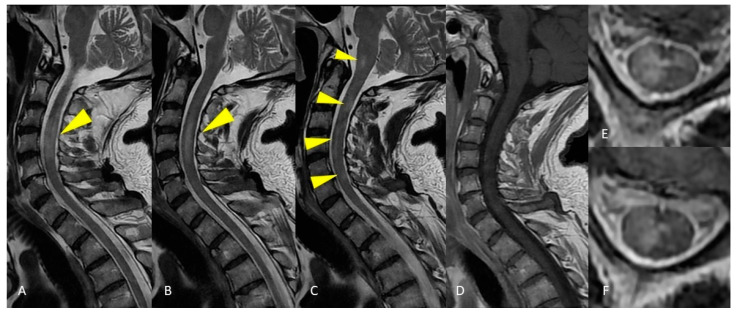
MRI of the cervical spinal cord. Sagittal T2-weighted images of the cervical spine show areas of patchy and long-segment (>1.5 vertebral body length) hyperintensity (A–C) without enhancement on the T1-weighted post-gadolinium acquisition (D). Axial T2-weighted image shows large hyperintensity involving half or more than half of the cross-sectional area of the spinal cord (E,F).
